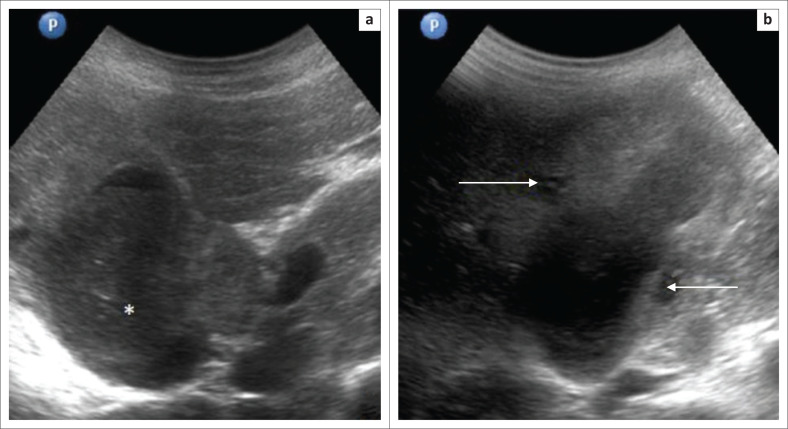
(a) Ultrasound abdomen revealing a distended gallbladder filled with echogenic sludge (asterisk), (b) with asymmetrical wall thickening and intramural hypoechoic nodules (arrows).
